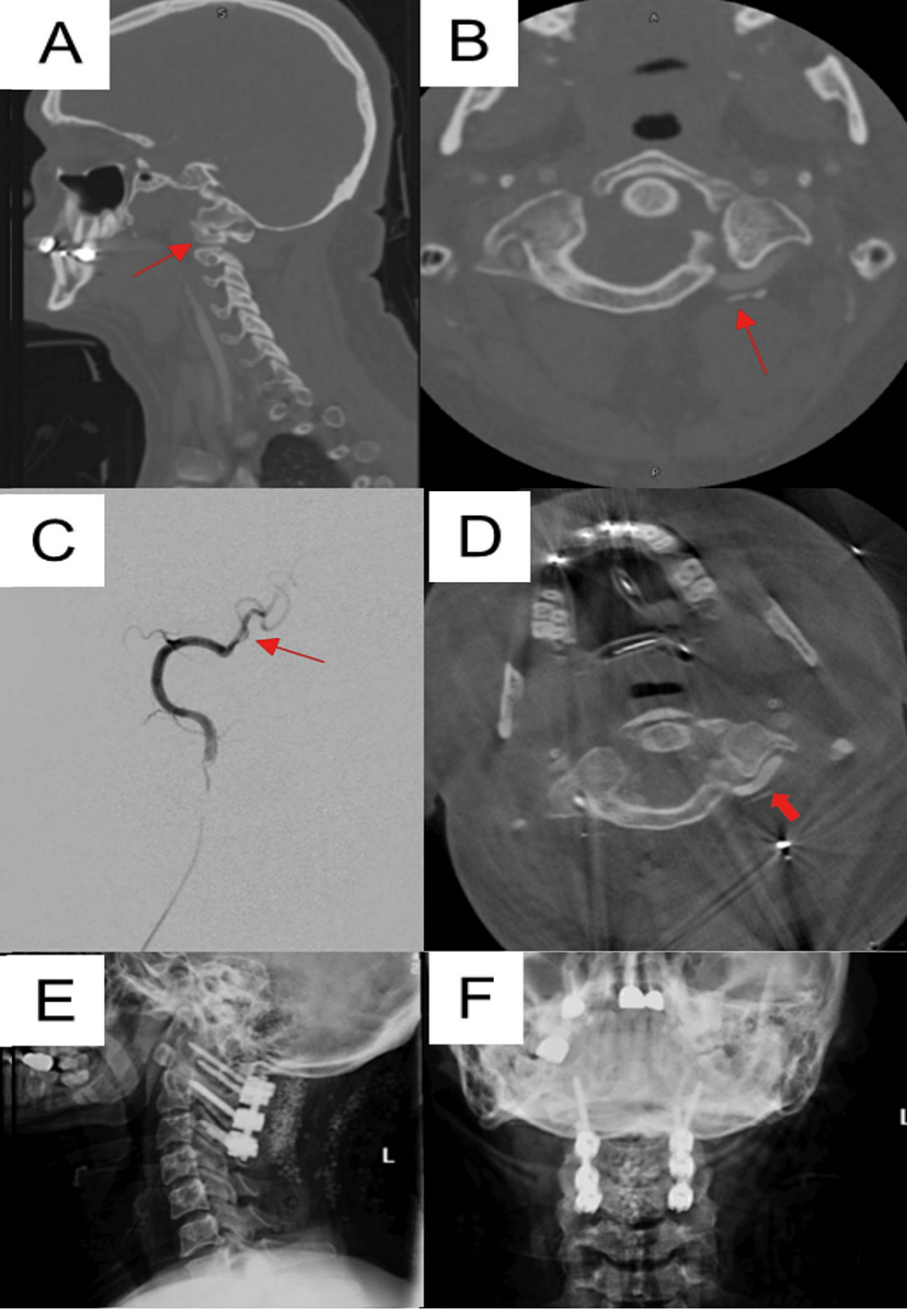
Trauma case A) Pre-operative sagittal CTA; B) pre-operative axial CTA; C) Intra-operative digital subtraction angiogram demonstrating catheter placement within the proximal right vertebral artery; D) Intra-operative computed tomography angiography (CTA) using O-arm (Medtronic, Minneapolis, MN) demonstrating the visualization of distorted vertebral arteries (red arrow); E) Post-operative lateral X-ray; F) Post-operative anteroposterior (A/P) X-ray.
